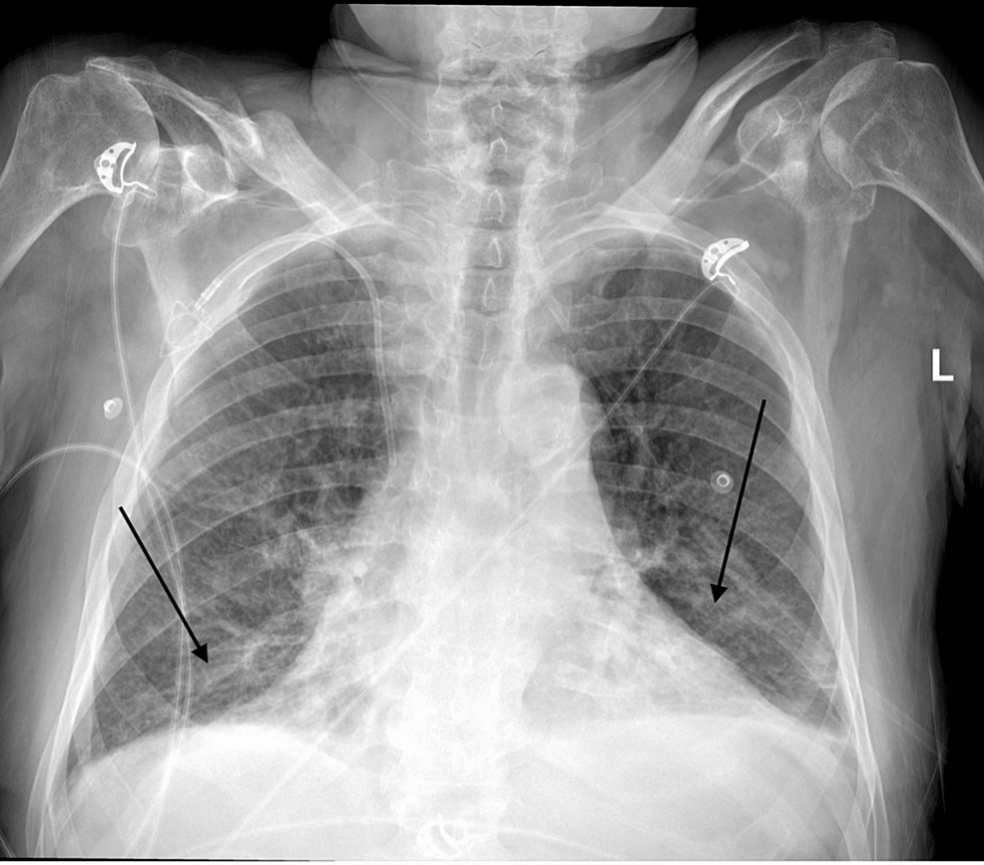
Follow-up chest X-ray The arrows on the follow-up chest X-ray indicate newly developed bilateral, bibasilar, parenchymal opacities.
